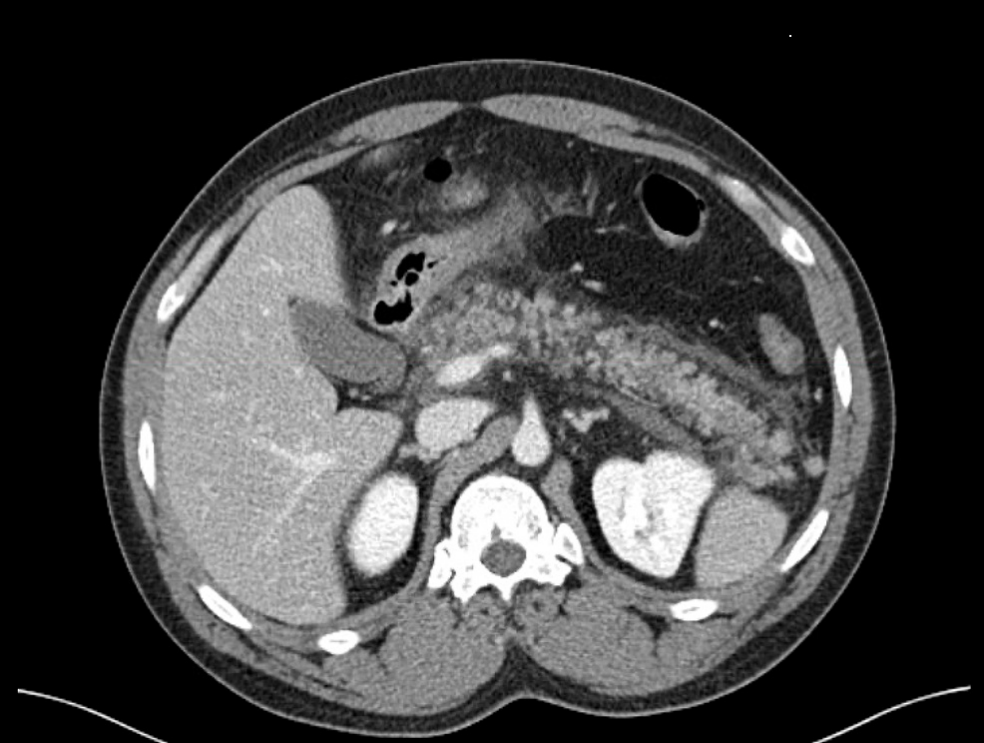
Initial CT scan of the abdomen CT scan of the abdomen with IV contrast demonstrated acute interstitial edema of the pancreas CT: computed tomography
